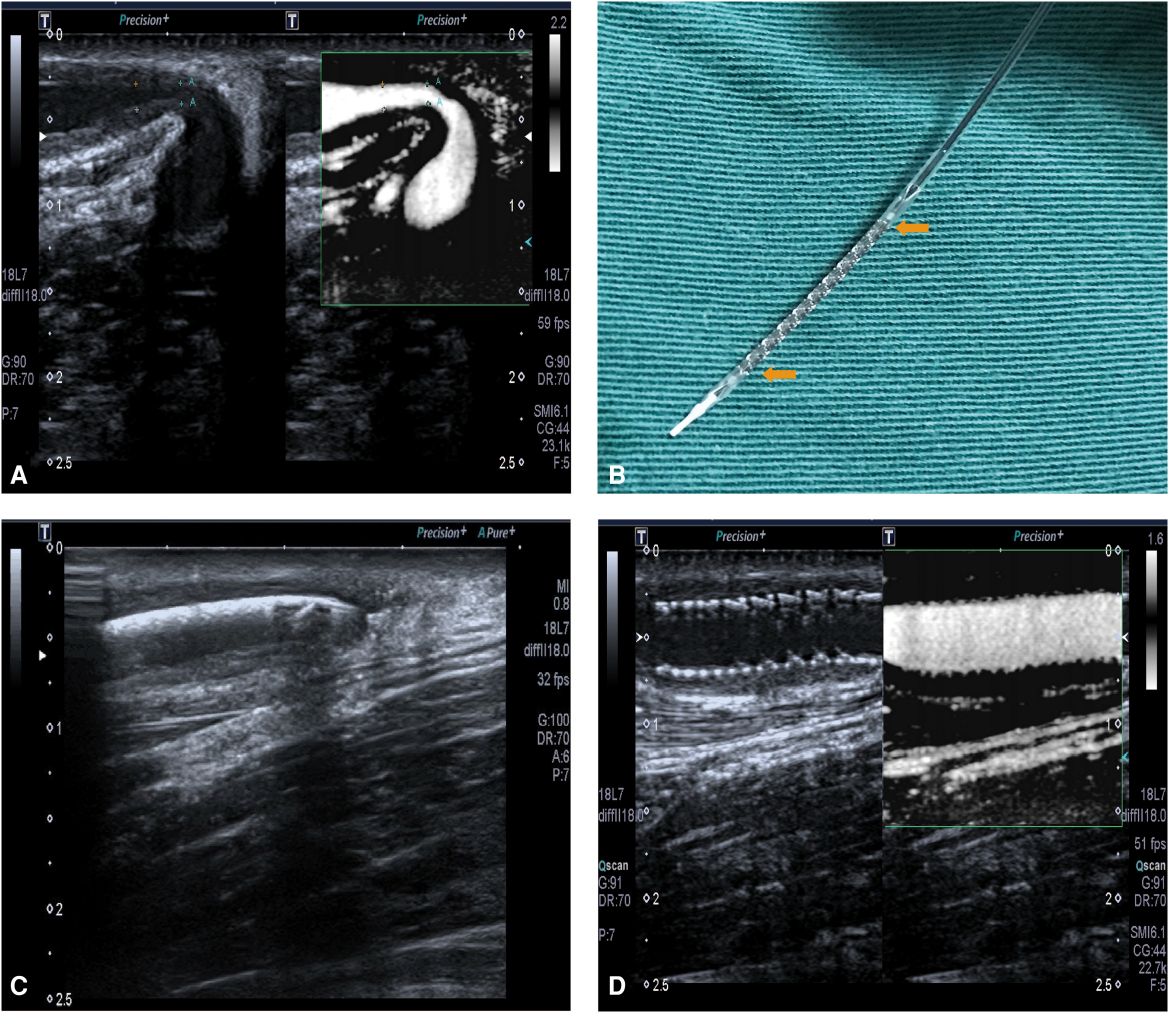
(A) The restenosis of right forearm autologous AVF on 23 May 2017. (B) RES. The yellow arrows show the main body of the stent, with a balloon inside it. (C) The ultrasound localization of RES which shows the RES was placed at the site of stenotic lesion. (D) The fully expanded RES, with a patent blood flow.
